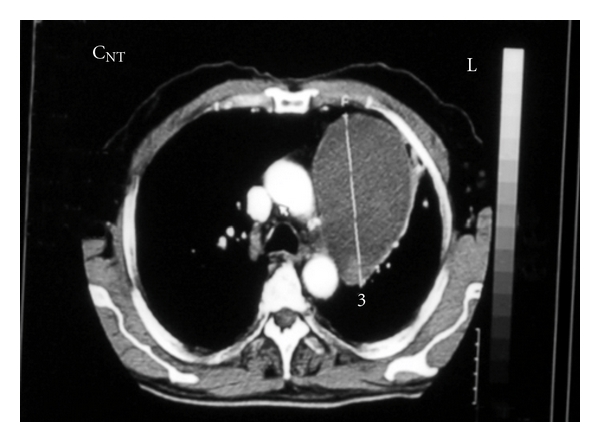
Computed tomography shows homogeneous cystic mass at the costosternal junction.
